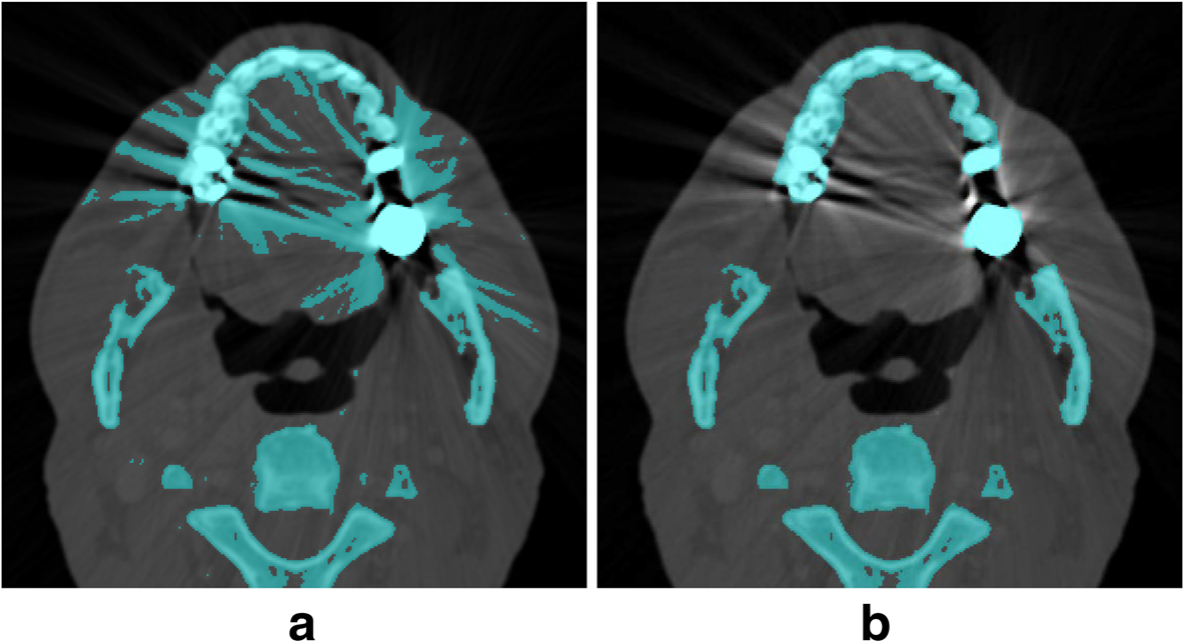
CT images of a patient with dental fillings (a) and that after application of a metal artifact reduction algorithm (b). Pixels in blue color were results of thresholding segmentation, aiming to separate bone from soft tissues. Metal artifacts contaminated the segmentation in the original images (a) while was removed in the image with metal artifact reduction algorithm (b)
